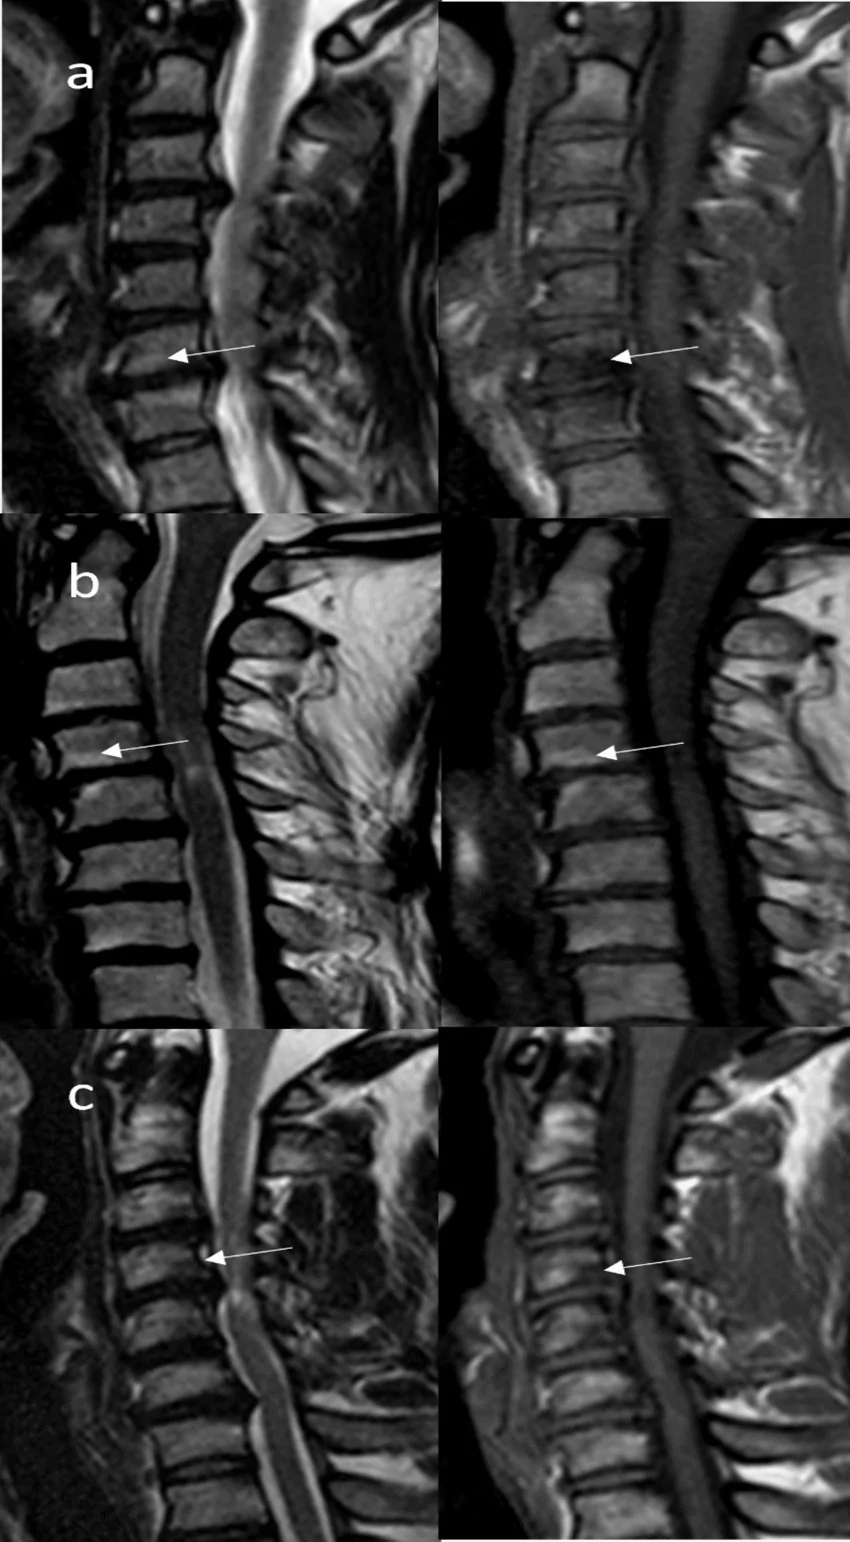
Representative cervical Modic changes. Mid-sagittal T2-weighted images (left column) and corresponding T1-weighted images (right column) showing (a) Modic type 1, (b) Modic type 2, and (c) Modic type 3 changes (arrows).
